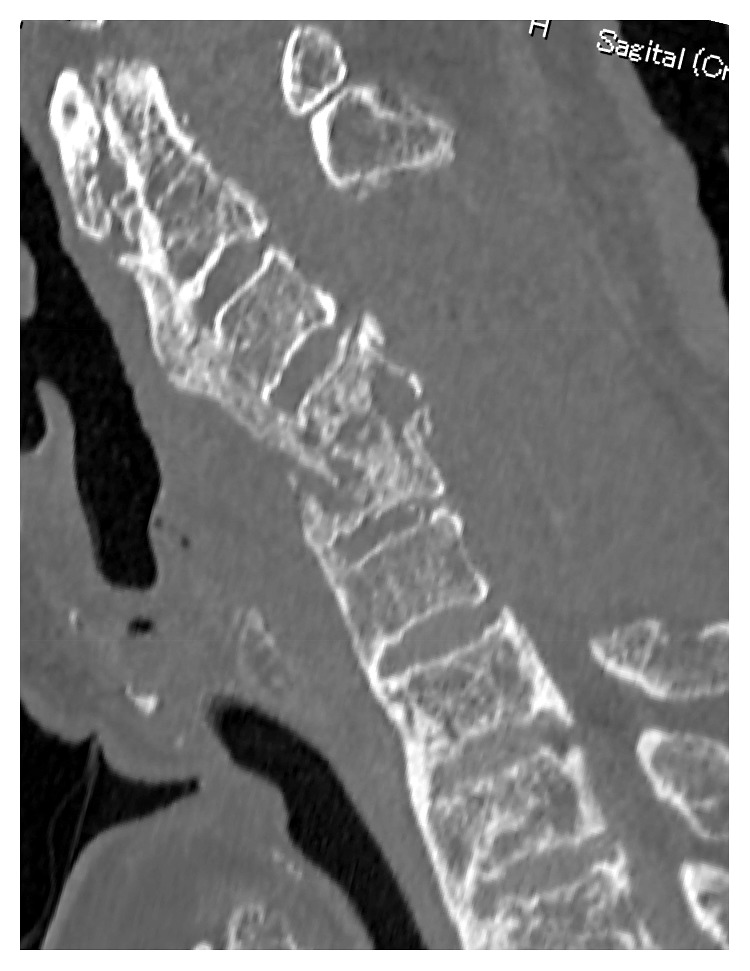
Sagittal CT showing an osteolytic and destructive change at the C4 and 5 vertebra and local kyphosis.
